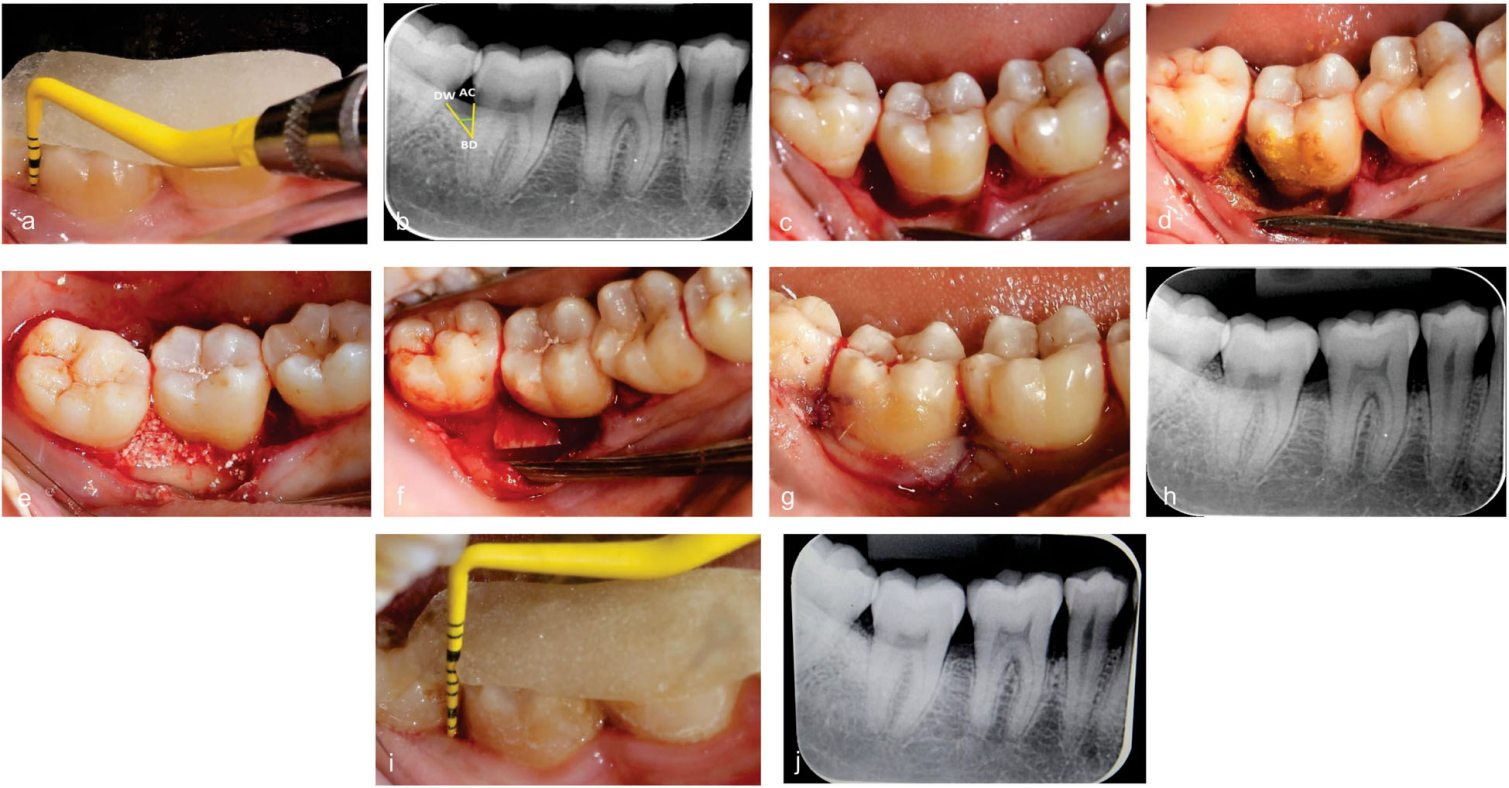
a Pre-operative clinical images with acrylic stent images with acrylic stent in place to measure the PPD, CAL in the region of distobuccal [7(7) mm] aspect of 47. b Preoperative RVG with intrabony defect in relation to distal aspect of 47. c Full thickness flap elevated in relation to 45, 46, 47 with simplified papilla preservation in relation to distal of 47 showing two wall defect from the CEJ to the base of the defect in relation to distobuccal aspect of 47 = 6mm. d Root conditioning done with tetracycline in relation to 47. e Defect site grafted with B-ostin HT(HA60 %:BTCP40 %) and (f) collagen membrane placed over the grafted area in relation to 47. g External vertical mattress suturing done with 4 − 0 vicryl sutures. h Immediate post-operative digital intra oral radiograph showing complete defect fill in relation to distal aspect of 47. i Six months post-operative clinical images with acrylic stent in place to measure the PPD, CAL in the region of distobuccal [2(3) mm] aspect of 47. j Six months post-operative digital intra oral radiograph in relation to distal aspect of 47 showing complete defect fill
